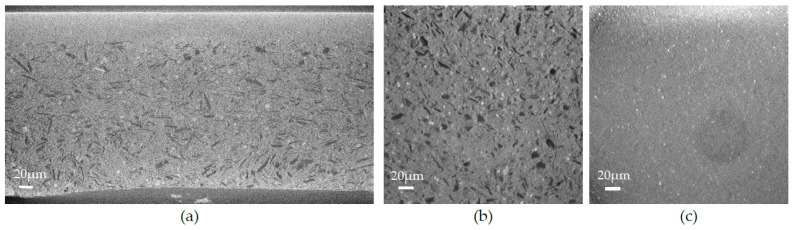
X-ray tomography images of the annealed sample: (a) cross-section along the *X*-axis; (b) cross-section of the anode along the *Y*-axis; (c) cross-section of the AFL along the *Y*-axis.
